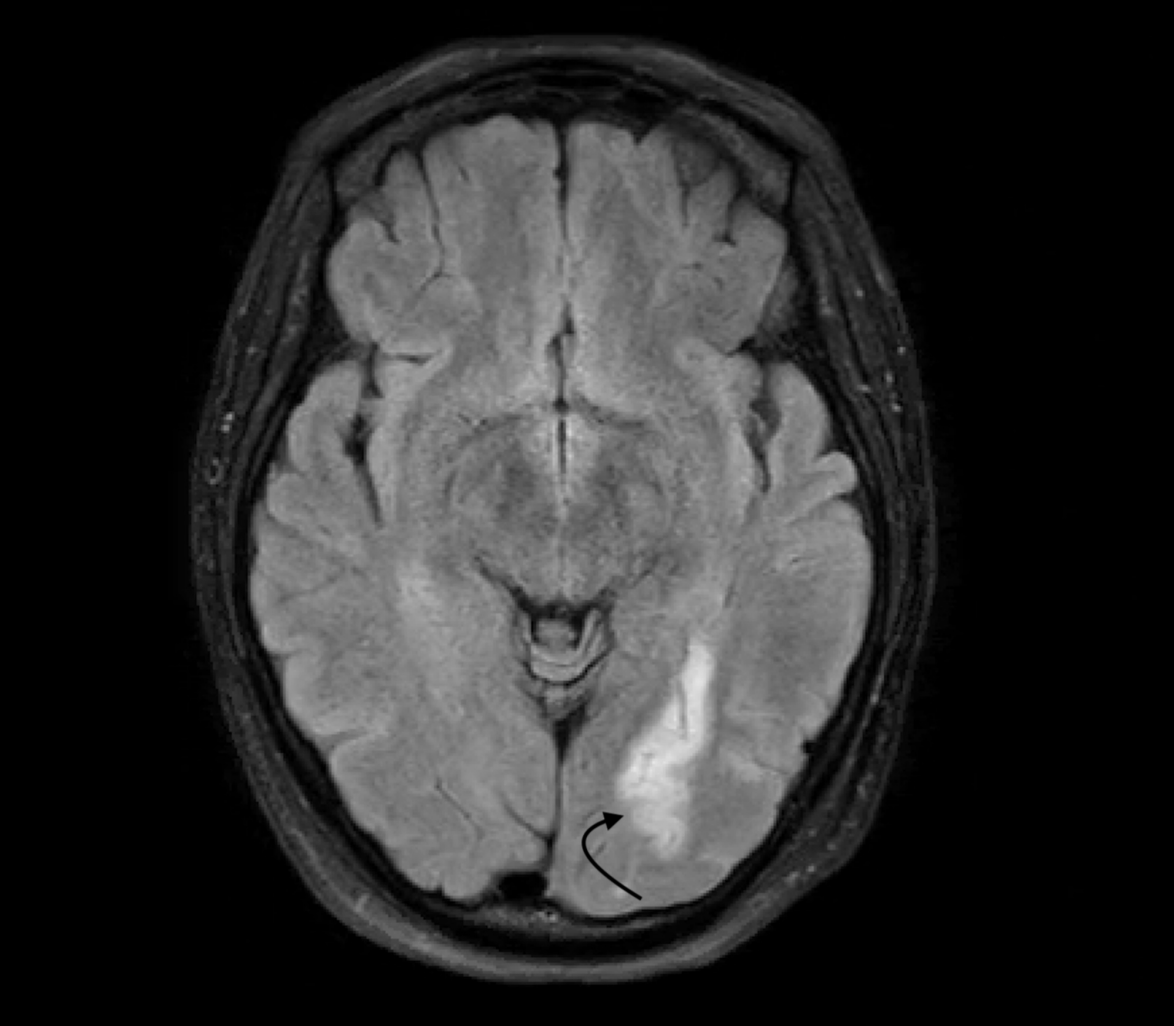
MRI of Brain With And Without Contrast An abnormal increase in signal density on FLAIR sequence consistent with an acute left posterior artery infarction
